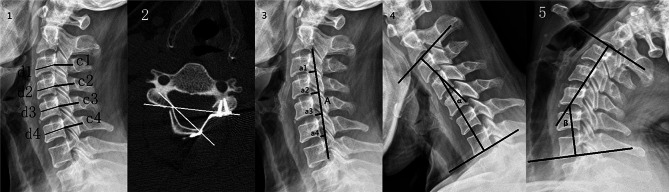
(1) Mean anterioposterior diameter = (c1 + c2 + c3 + c4)/4. Mean Pavlov`s ratio = (c1/d1 + c2/d2 + c3/d3 + c4/d4)/4. (2) Measurement of open angle on CT image. (3) Lateral radiograph showing evaluation of the cervical curvature index (CCI) with the Ishihara method: CCI=(a1 + a2 + a3 + a4)/A. (4, 5) The cervical range of motion (ROM) was measured on the flexion-extension radiograph. ROM = α + β
